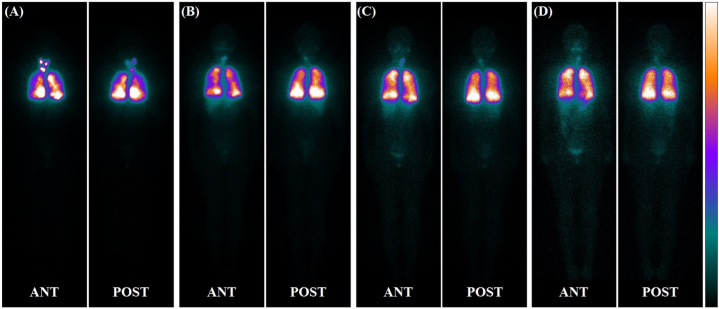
Iodine-131 treatment for DTC pulmonary metastases achieved SIR (the Rx-WBS imaging showed diffuse distribution) A 19-year-old female presented with follicular carcinoma of the thyroid gland in both lobes. Chest CT: The maximum diameter of the pulmonary metastases was 0.82 cm. Four times ^131^I treatments at a activity of 5.55GBq/time. Whole body anterior (ANT) and posterior (POST) plane imaging after ^131^I treatment. Imaging after initial ^131^I treatment (A): ^131^I uptake in the thyroid bed and pulmonary metastases showing diffuse ^131^I uptake. Imaging after the 2nd_4th treatment(B,C, and D): Faint uptake in the thyroid bed; pulmonary metastases showed diffuse ^131^I uptake, and the degree of ^131^I concentration was gradually reduced; TgAb value negative; sTg value was 605.5 ng/mL, TSH-suppressed Tg values were 47.06/33.80/21.57 and 18.52 ng/mL after 1st-4th ^131^I treatment, respectively.
